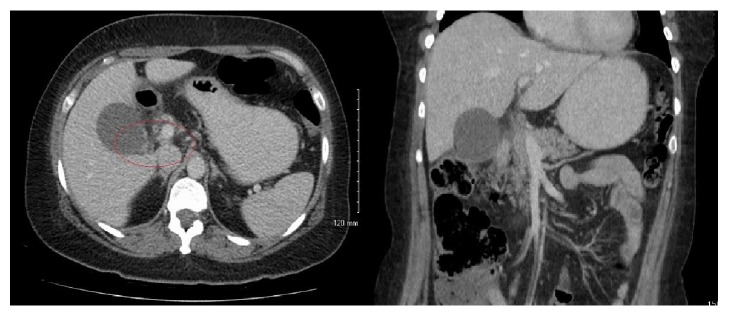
CT abdomen pelvis day 2: periportal and pericholecystic fat stranding with hyperaemia of the extrahepatic bile duct and adjacent stranding. Findings are suspicious for an ascending cholangitis and cholecystitis.
